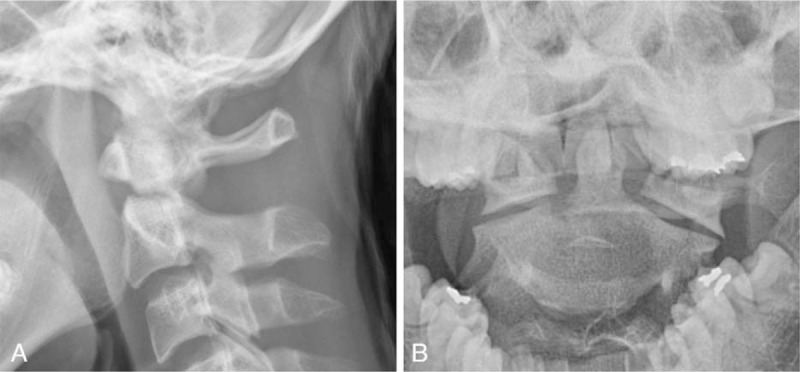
Lateral radiograph (A) and open mouth view (B) showing successful reduction of C1–2 posterolateral dislocation with type II dens fracture by skeletal traction.
